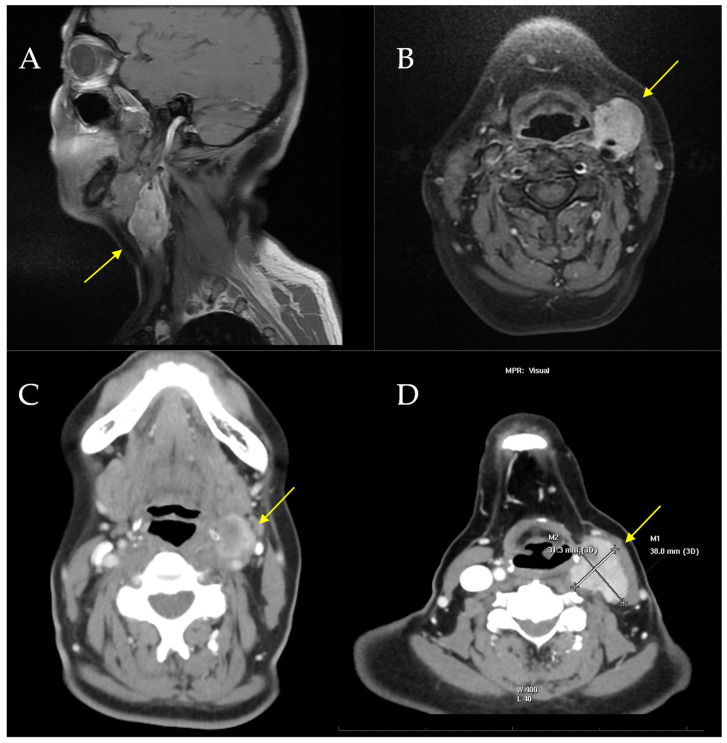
Extraenteric malignant gastrointestinal neuroectodermal tumor involving the soft tissues of the neck in a 58-year-old woman: (A) Sagittal MRI image at diagnosis. (B) Axial MRI image of the tumor at diagnosis. (C) Axial CT image in which vascular invasion by the tumor is observed at diagnosis. (D) Tumor measurements in the preoperative CT scan.
